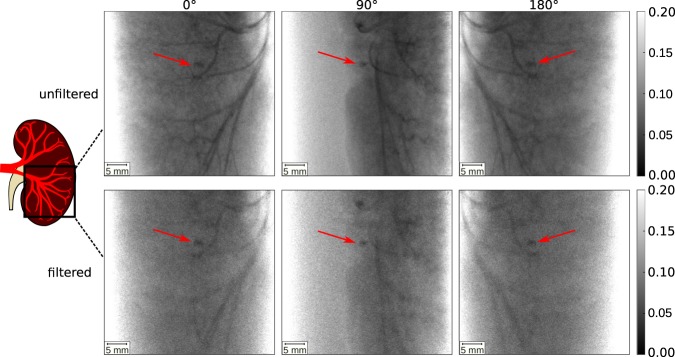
MuCLS X-ray projection images filtered and unfiltered CTs at projection angles 0°, 90° and 180°. The kidney stone is indicated by a red arrow. In the unfiltered projection images, the iodine filled blood vessels are clearly visible together with the kidney stone. In the filtered projection images, the X-ray attenuation of the iodine contrast agent is reduced, yet still the differentiation of iodine and the kidney stone is difficult. The gray scales of the projection images show the relative transmission of the X-ray beam.
